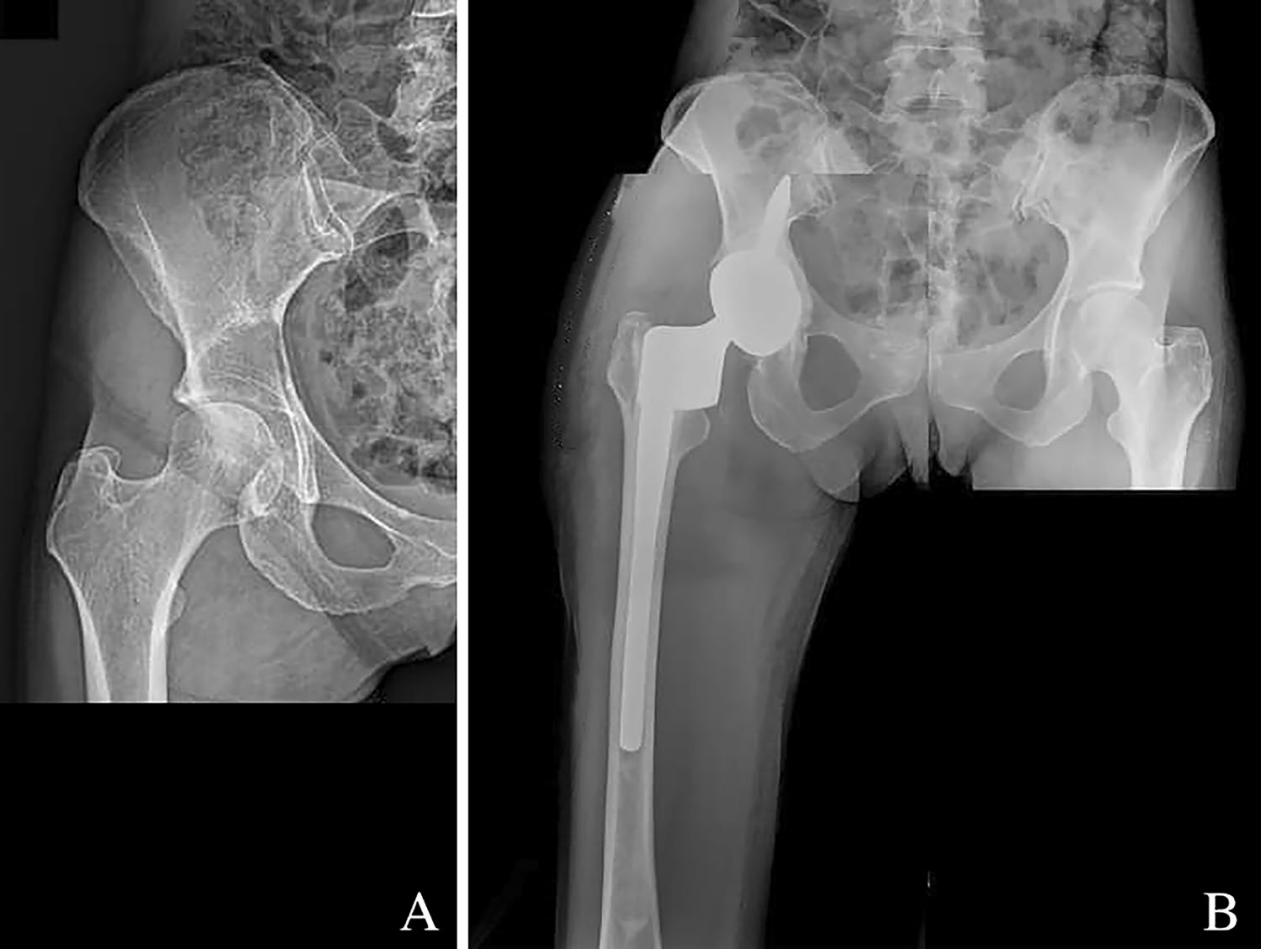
A Preoperative X-ray showing a consistent osteolysis caused by a metastasis from breast cancer in a 55-year-old woman; B post operative X-ray showing the use of a SAC acetabular prosthesis used to reconstruct the hip after curettage of the cavity
